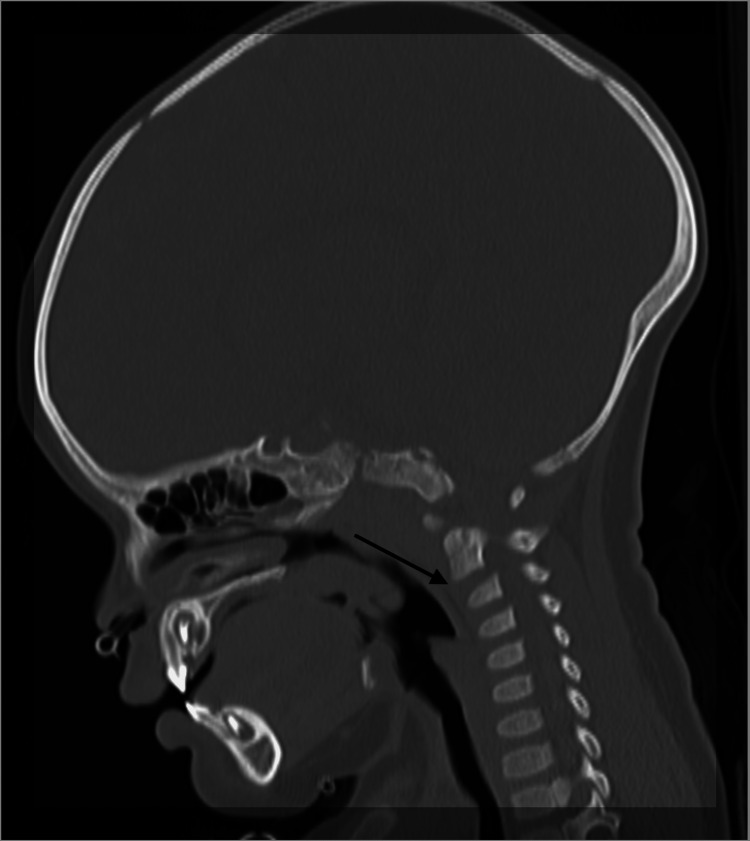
Sagittal cut section of the head and neck CT scan showing mild listhesis at the C2/C3 level. CT: computed tomography
